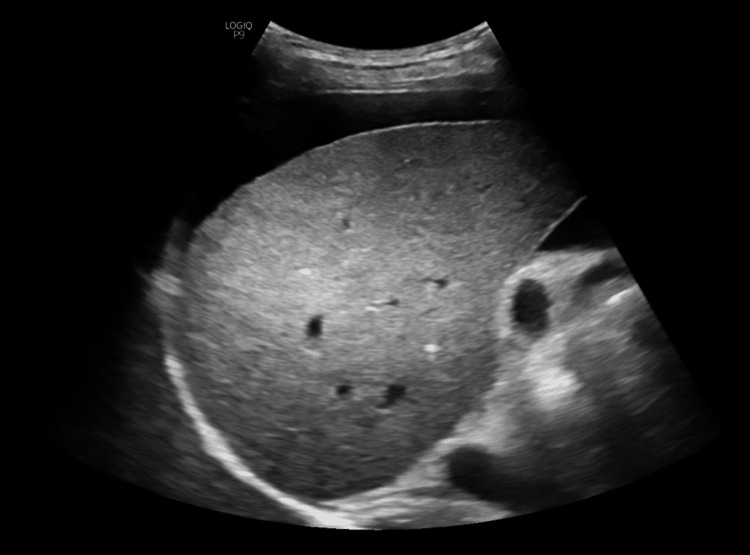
Ultrasound abdomen of case 5. The image reveals moderate ascites, a shrunken liver with coarsened echotexture, and an irregular surface.
